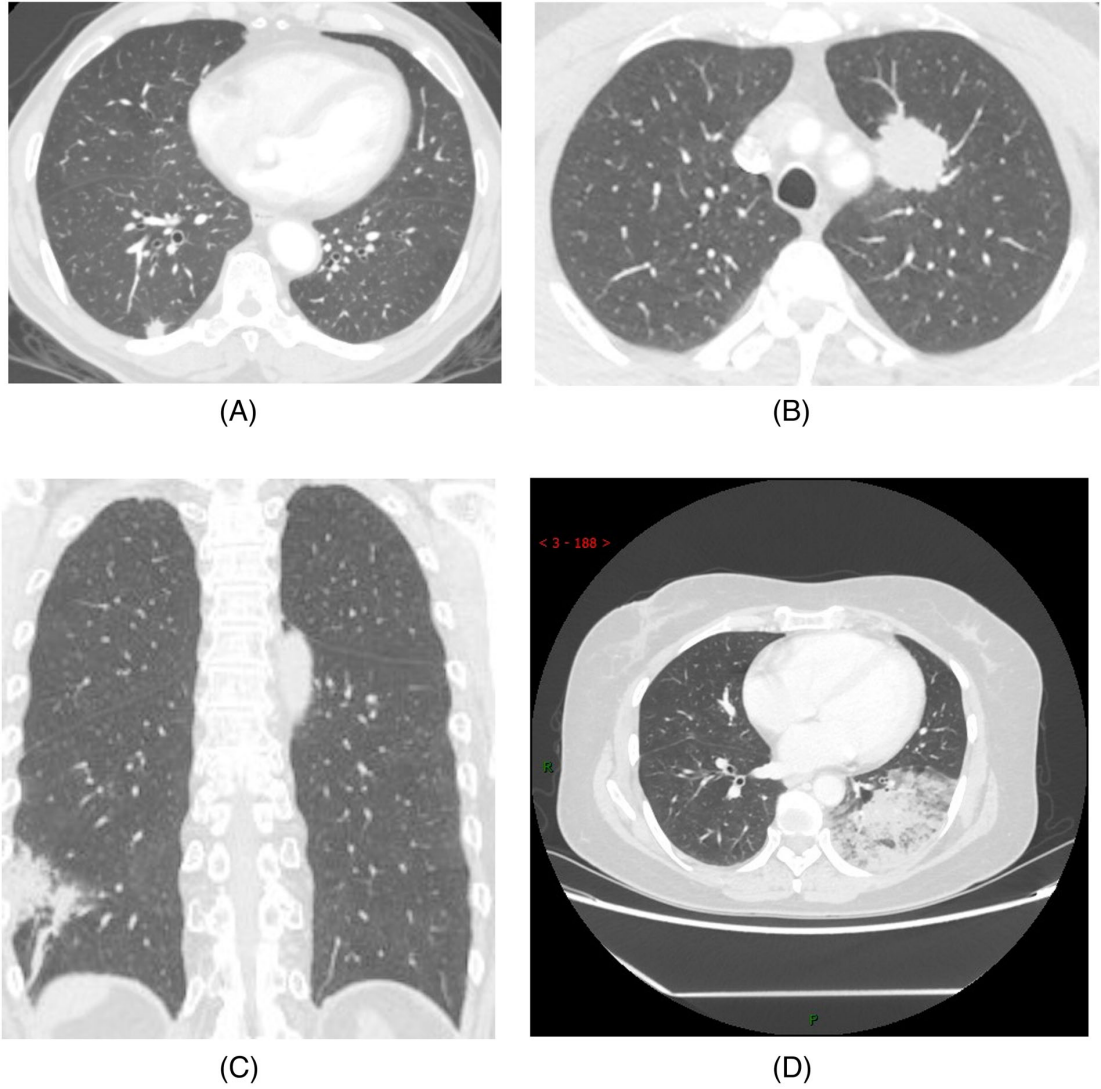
The CT imaging classification of invasive mucous adenocarcinoma (IMA). (A) CT imaging of nodular‐type IMA (*d* < 3 cm), (B) mass‐type IMA (*d* ≥ 3 cm), (C) patch‐type IMA, and (D) large consolidative‐type IMA.
